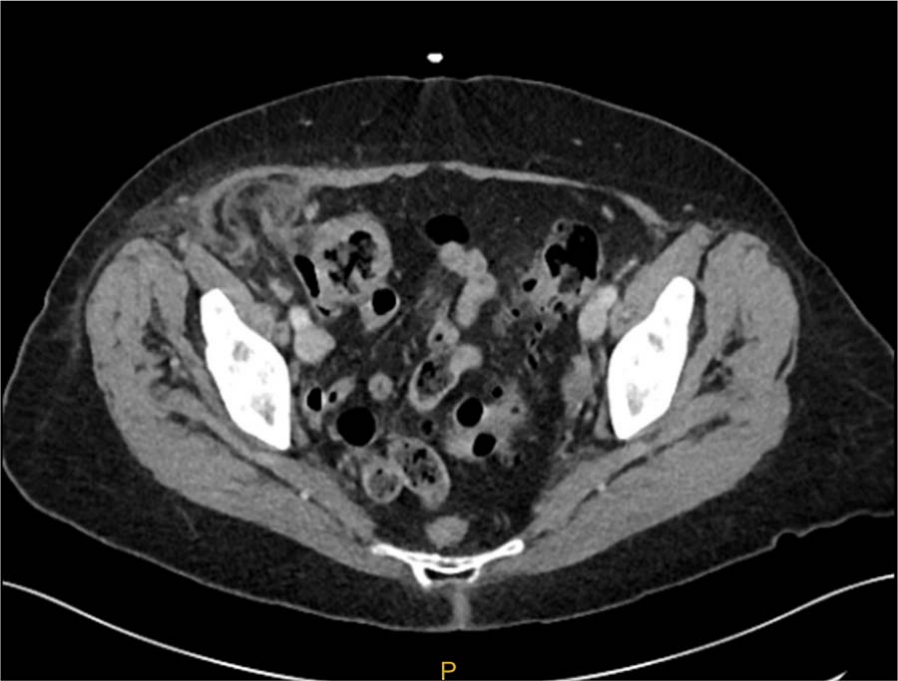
Preoperative CT image of right inguinal hernia. It is difficult from the images to determine if the hernia sac contains an appendix.
